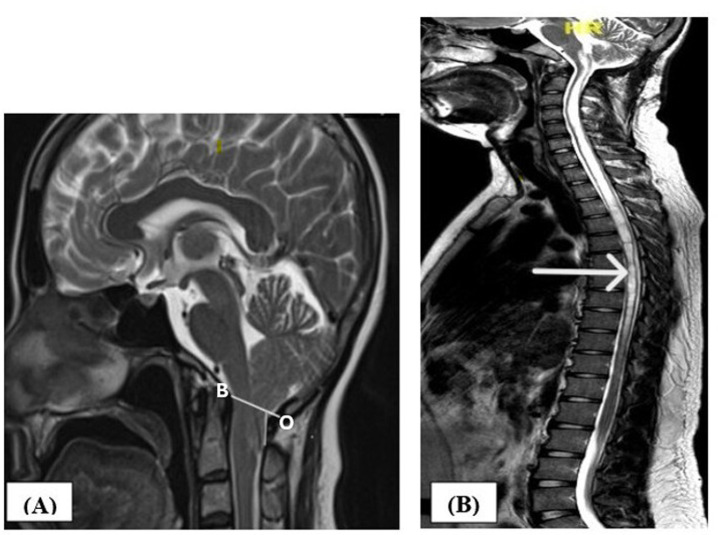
A. Magnetic resonance imaging (MRI) scan of a Chiari malformation type 1 (CM1) patient showing tonsillar herniation extending more than 5 mm below the foramen magnum (the white line denotes the McRae line connecting the basion and opisthion). B. Sagittal T2-weighted MRI image demonstrating cervical-thoracic syringomyelia in a CM1 patient, as indicated by the white arrow.
